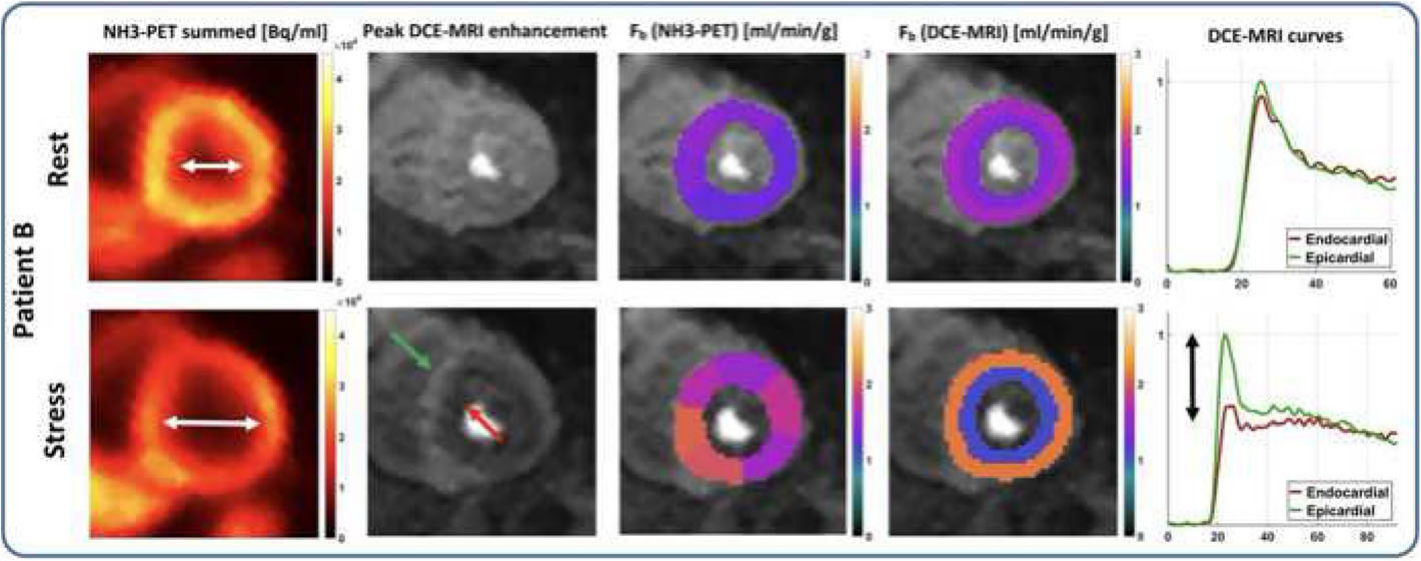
Hybrid PET/MRI myocardial perfusion assessment in a patient with hypertrophic cardiomyopathy. The apparent dilatation of left ventricular cavity during adenosine challenge (first column) is caused by a stress-induced subendocardial hypoperfusion as demonstrated by MRI MPI (column two to five). (Reprinted with permission from Kunze KP, Nekolla SG, Rischpler C, et al. Myocardial perfusion quantification using simultaneously acquired 13NH3-ammonia PET and dynamic contrast-enhanced MRI in patients at rest and stress. Magn Reson Med. 2018;00:1–14. 10.1002/mrm.27213)
